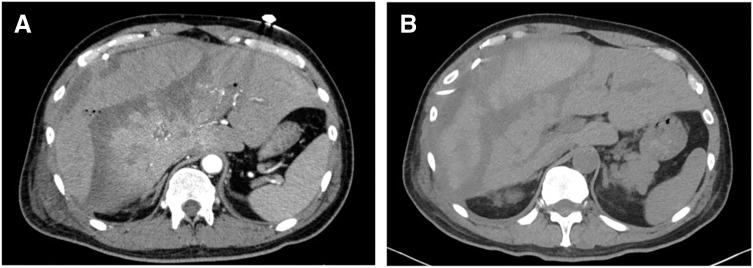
Follow-up contrast-enhanced CT. (A) Contrast-enhanced CT performed 3 days after TAE shows the disappearance of contrast extravasation, indicating successful hemostasis. (B) Follow-up CT on day 21 demonstrates regression of the subcapsular hematoma and hepatic infarction. TAE, transarterial embolization
